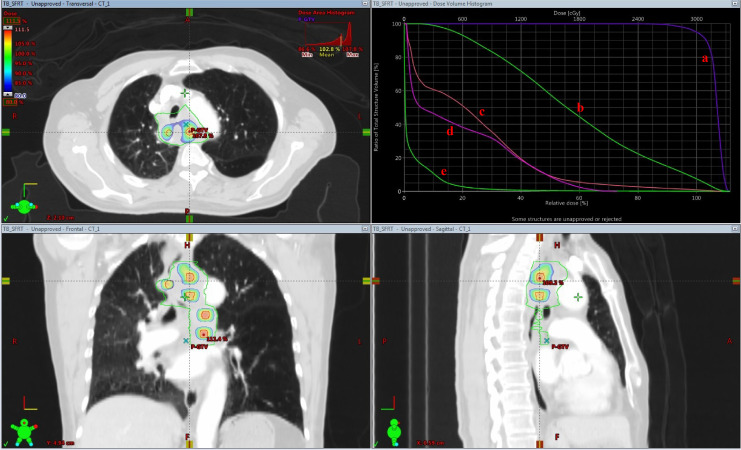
Dose distribution in target volume: the relative positions of high-dose regions (peaks) and GTV on axial, sagittal, and coronal CT images, and the corresponding DVH curves: Curve (a) DVH curve of the planning target volume (PTV), namely the SFRT target volume; curve (b) DVH curve of the entire solid tumor; curve (c) DVH curve of the esophagus; curve (d) DVH curve of the trachea; curve (e) DVH curve of the body.
